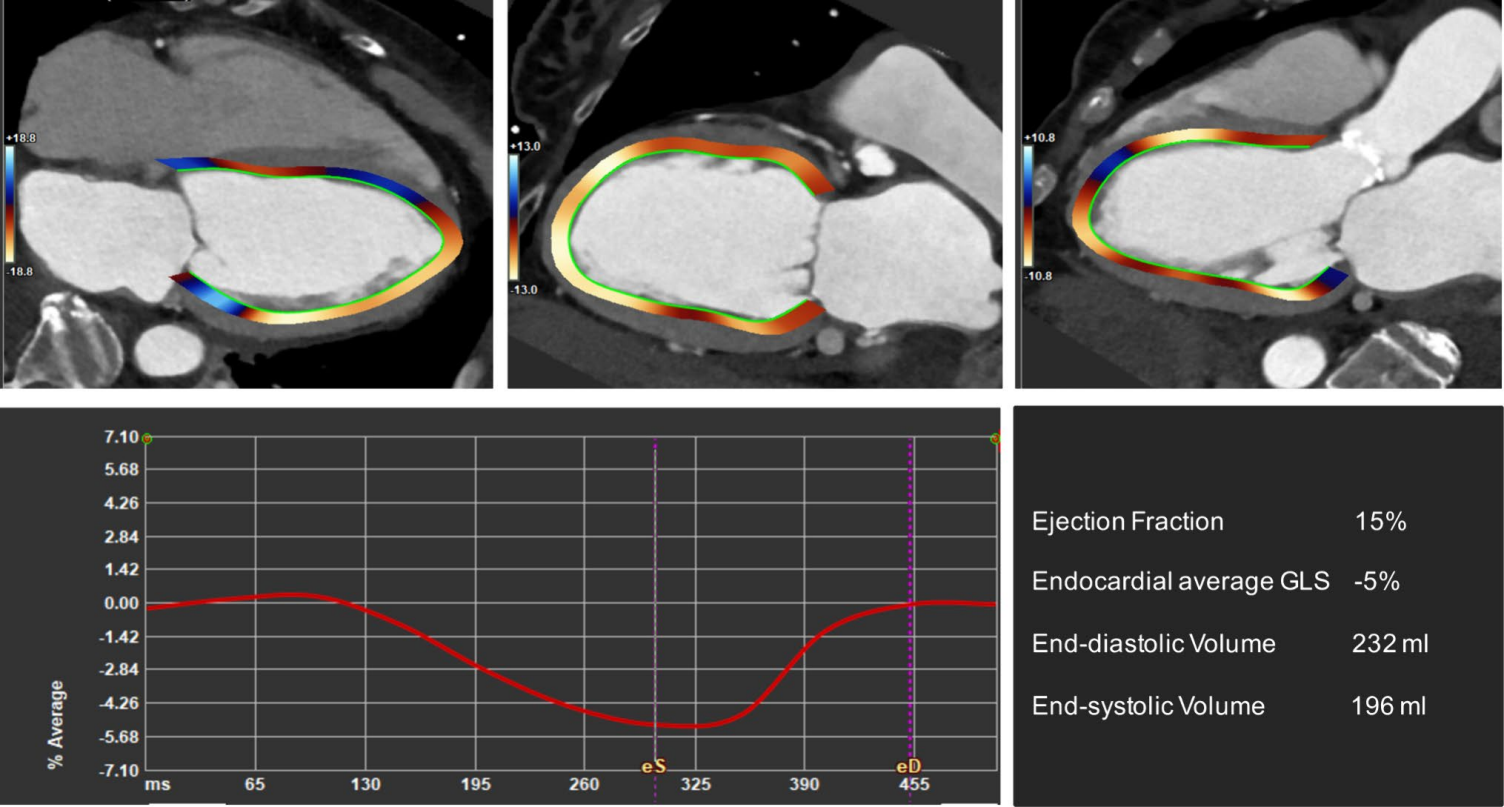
4D CT derived Global Longitudinal Strain (GLS). 74 year-old-female patient with severe aortic stenosis (see aortic calcifications in 3-chamber view, top line). Positive and negative endocardial end-systolic GLS values are marked in blu and red in the long-axis view (top line). The average GLS-time curve is presented (bottom left) with the prinicpal functional and volumetric parameters derived from the analysis (bottom right) showing abnormal average GLS (− 5%), strongly reduced ejection fraction (15%), and a severely dilated left ventricle
